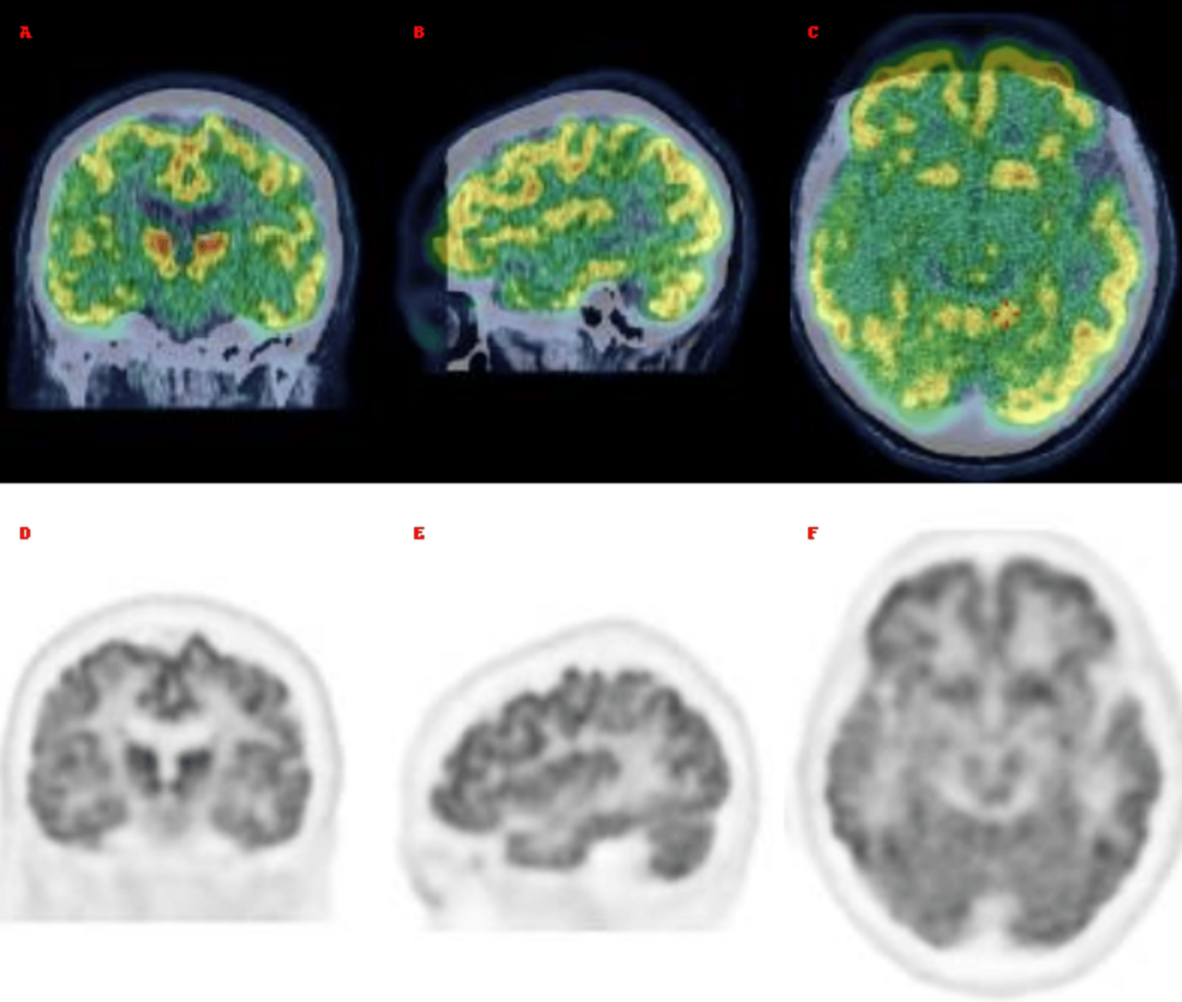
PET of the brain normal (A–C) Fused 18F-FDG PET/CT brain slices in coronal, sagittal, and axial planes, showing normal metabolic distribution. (D–F) Corresponding standalone 18F-FDG PET slices in the same planes, also demonstrating preserved metabolism. 18F-FDG PET/CT: 18F-Fluorodeoxyglucose positron emission tomography/computed tomography.
